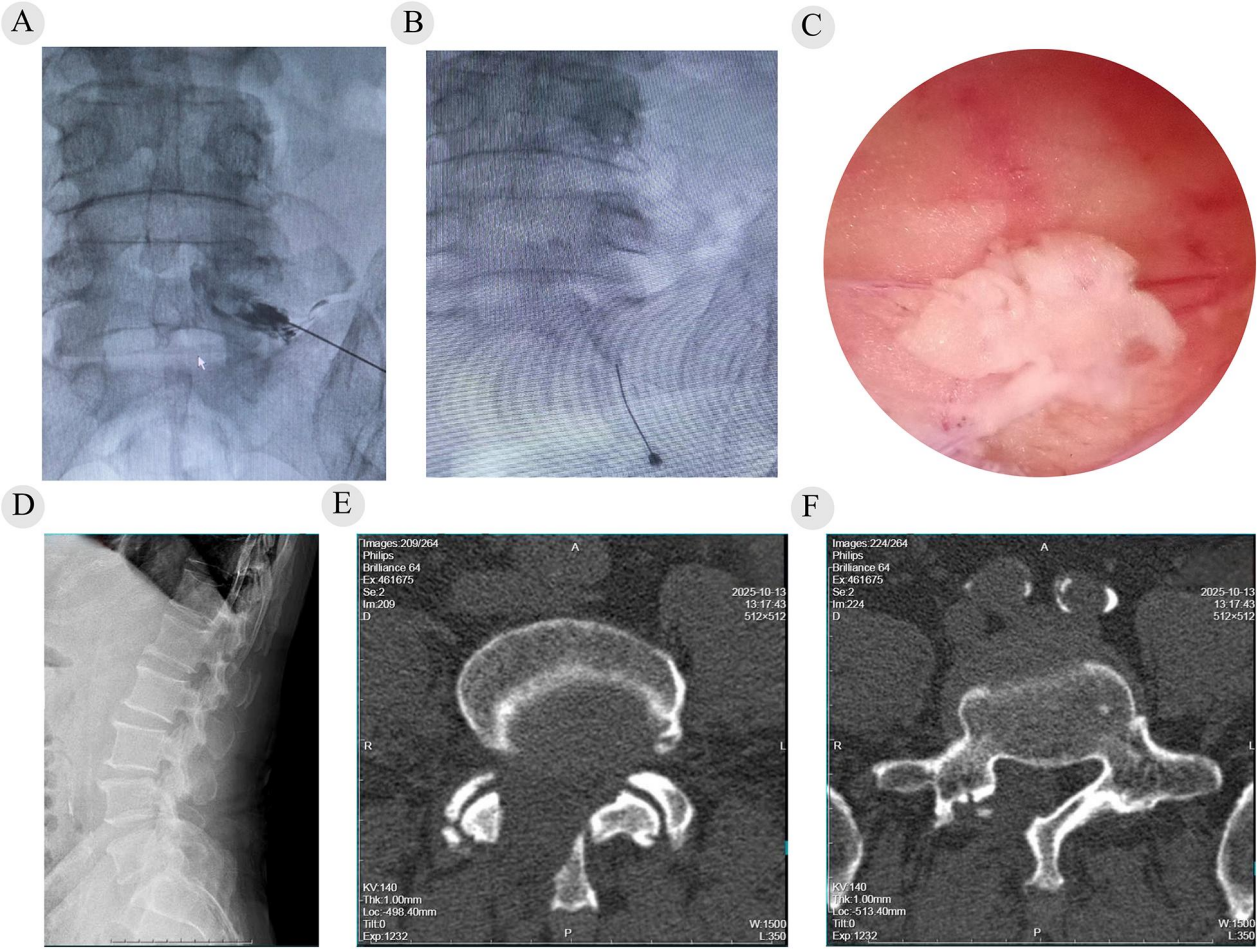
Intraoperative and postoperative findings. (A) Anteroposterior fluoroscopic view during SNRB at the L5 foramen, demonstrating contrast opacification outlining the L5 nerve root. (B) Fluoroscopic view during SNRB at the S1 sacral foramen, showing contrast spread along the S1 nerve root. (C) Intraoperative endoscopic (arthroscopy-assisted) view during Stage II L5/S1 decompression, demonstrating excessive posterior epidural fat. (D) Postoperative lateral lumbar radiograph. (E,F) Postoperative axial CT images at L4/5 and L5/S1, respectively, demonstrating adequate decompression. Written consent for publication was obtained.
